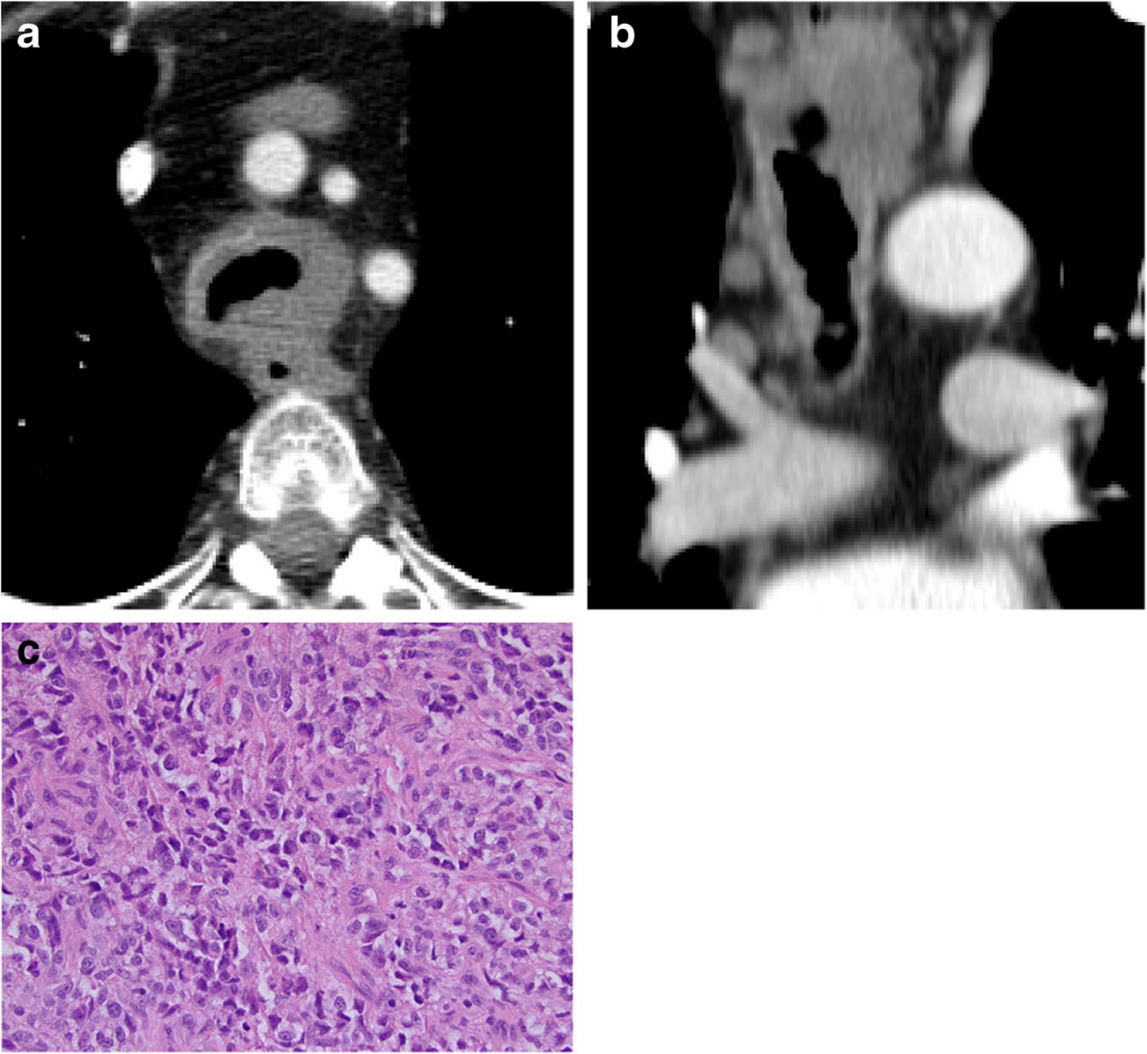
Sixty-eight-year-old man with a axial and b coronal CT images demonstrating soft tissue thickening, eccentric luminal protrusion, and infiltrative changes of the adjacent mediastinal fat. c Pathology reveals malignant cells that are positive for S100, HMB45 and Melan-A compatible with metastatic melanoma
